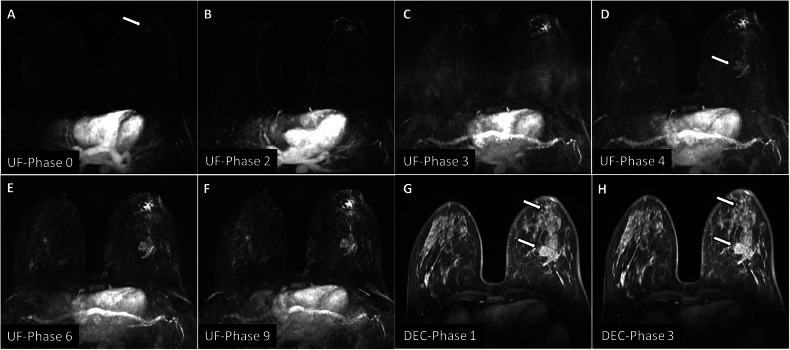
An example of how ultrafast MRI can increase lesion visibility compared to the conventional DCE-MRI. A–F Sequentially subtracted ultrafast MIP images. A A left retro-areolar irregular spiculated mass first appeared at phase 0 (arrow). D A second irregular mass first appeared at phase 4 (arrow), seen at the lower outer quadrant of the left breast. G, H The corresponding conventional DCE-MRI at phases 1 and 3, respectively, shows that the breast is dense with marked BPE obscuring the two masses (arrows)
